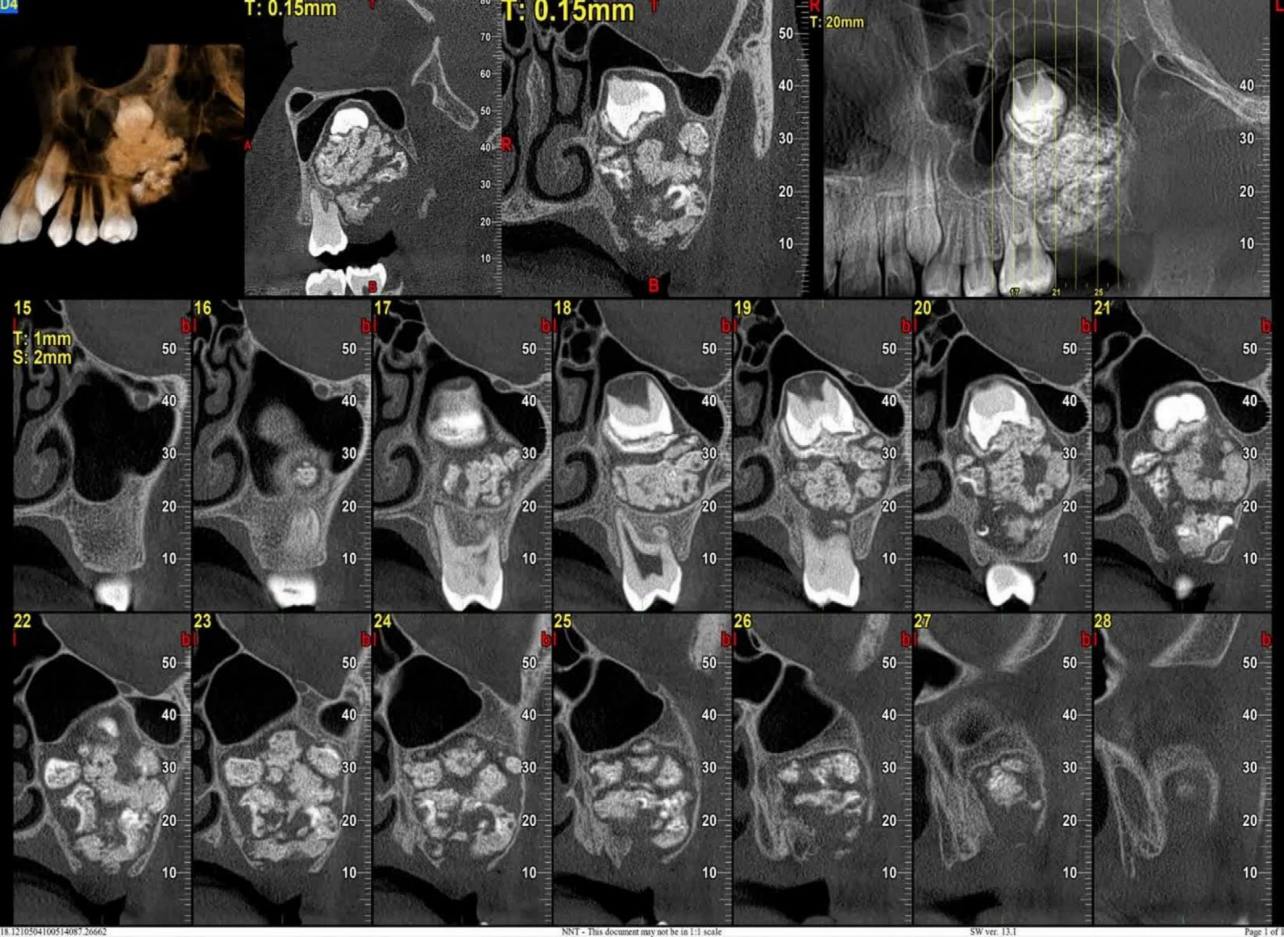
CBCT analysis using reformatted panoramic, sagittal, and 3D transparent views reveals a well‐circumscribed, mixed pericoronal lesion in the posterior region of the left maxilla.
